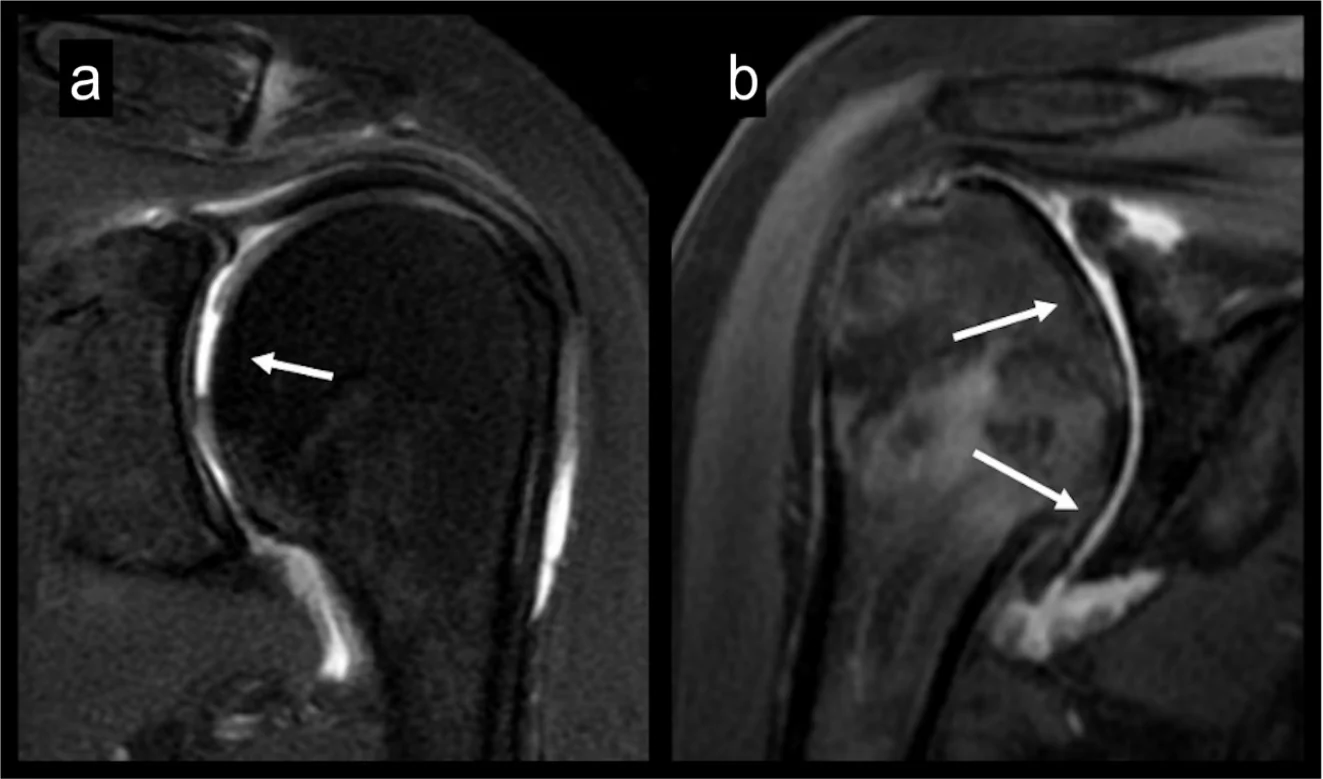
MRI findings of focal versus dif-fuse glenohumeral chondral lesions.
